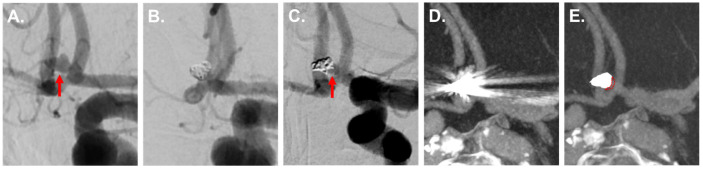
An 84-year-old patient with an aneurysm of the anterior communicating artery in the initial DSA ((A) red arrow) with consecutive treatment via endovascular coiling (B). Six months later, DSA control revealed a reperfusion at the aneurysm base ((C) red arrow). The aneurysm was not assessable in iMAR− FD-CTA due to severe metal artifacts (D). Thanks to a significant metal artifact reduction, iMAR+ FD-CTA also demonstrated the basal aneurysm reperfusion in accordance with the DSA ((E) marked with red dotted line).
